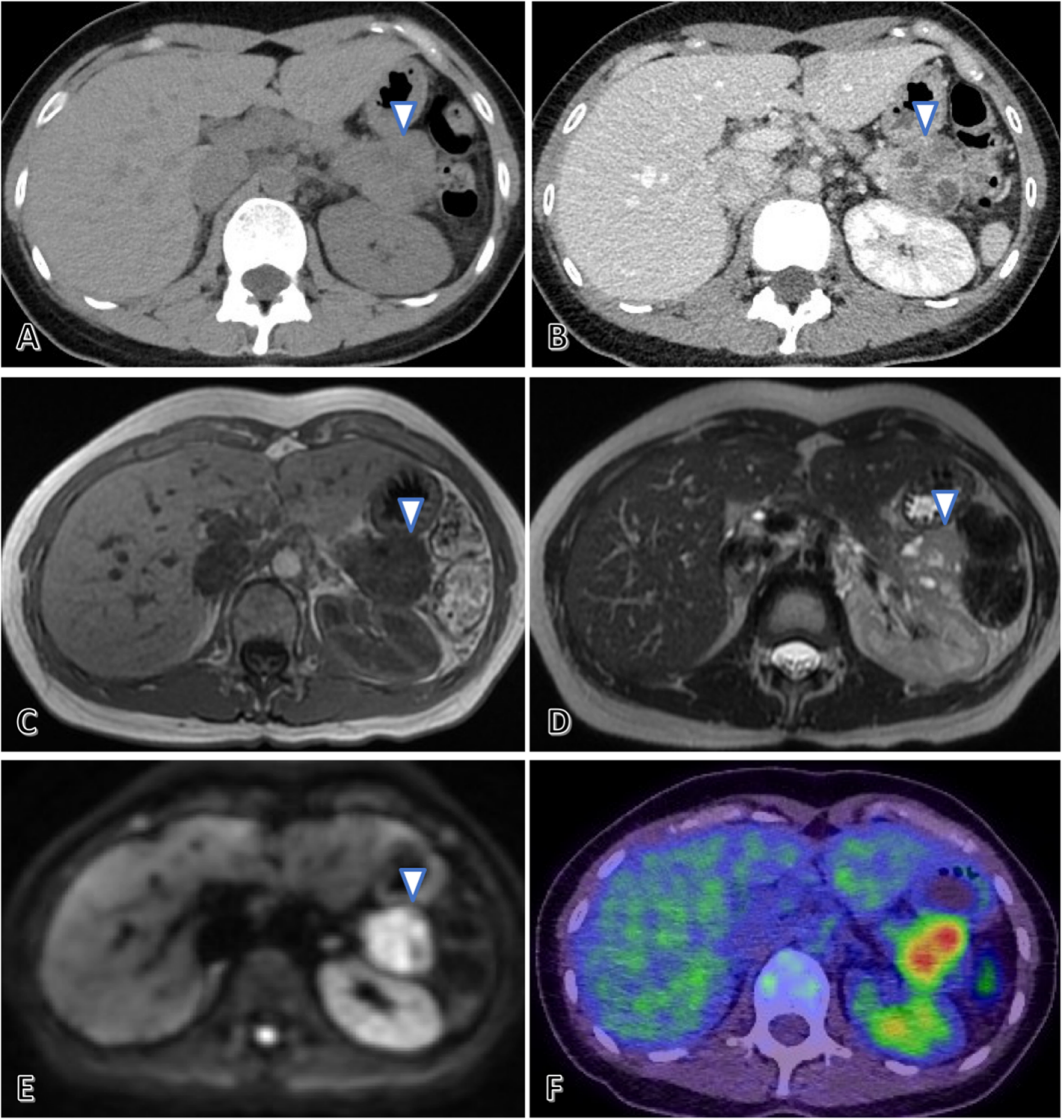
Radiological findings of the pancreatic tail tumor. Non-contrast abdominal computed tomography (CT) reveals a large, 35-mm tumor in the pancreatic tail without calcification (arrow) (a). Contrast-enhanced CT shows that the tumor had heterogeneous contrast enhancement without bleeding (arrow) (b). Abdominal magnetic resonance imaging reveals that the pancreatic tail tumor (arrow) presented as a low signal area on the T1-weighted image (c), a slightly hyperintense tumor containing multiple cystic components on the T2-weighted image (d), and a high signal area on the diffusion-weighted image (e). Positron emission tomography CT indicates abnormal fluorine-18 fluorodeoxyglucose accumulation only in the pancreatic tail tumor (f)
